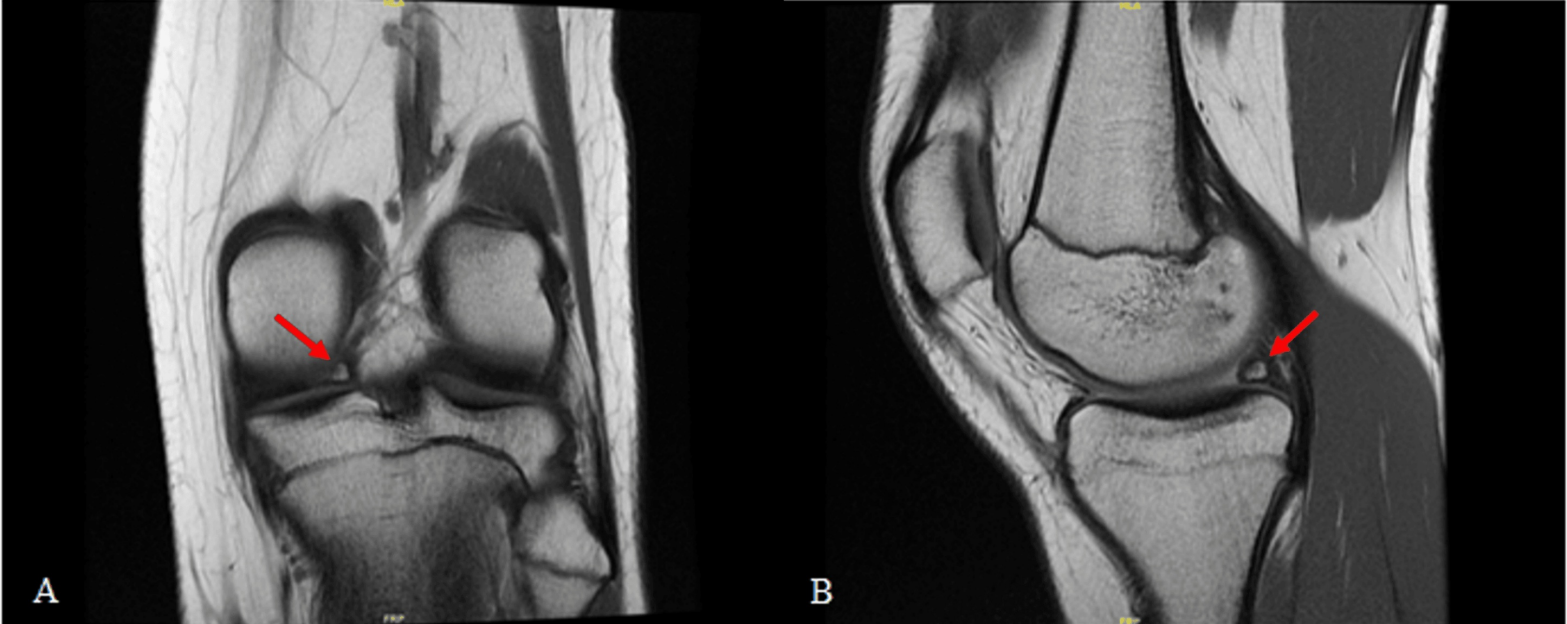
MRI of the left knee suggestive of an intra-meniscal ossicle (arrow) in the posterior horn of the medial meniscus in both coronal (A) and sagittal (B) views.
